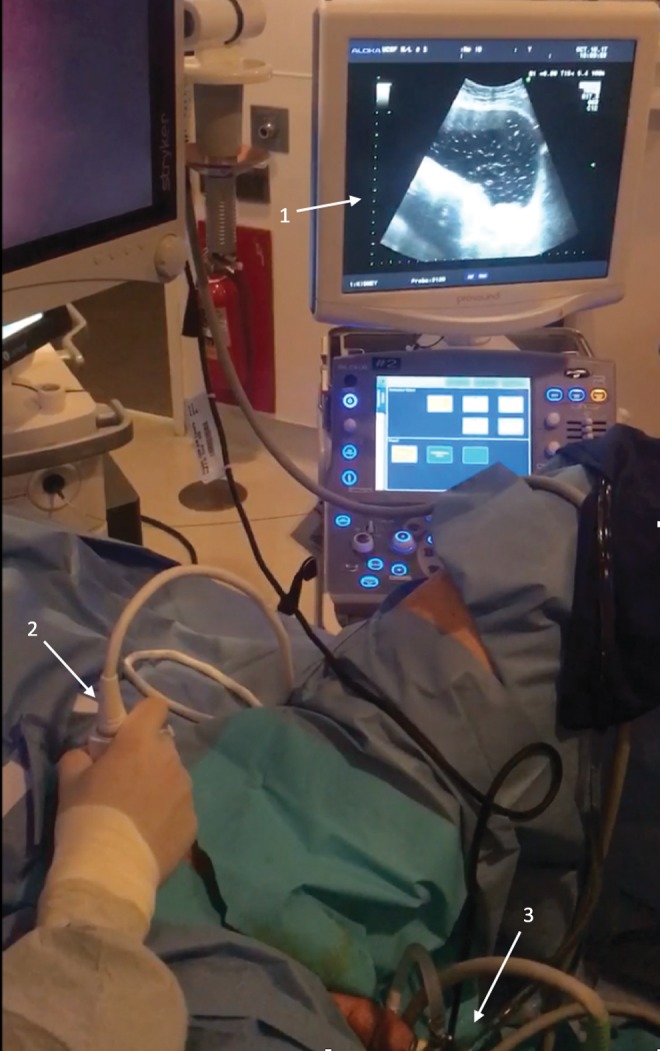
Arrangement of ultrasound machine with use of convex abdominal transducer probe under B-mode ultrasound to observe bladder in sagittal view. 1 = ultrasound monitor and console; 2 = ultrasound probe; 3 = resectoscope.
